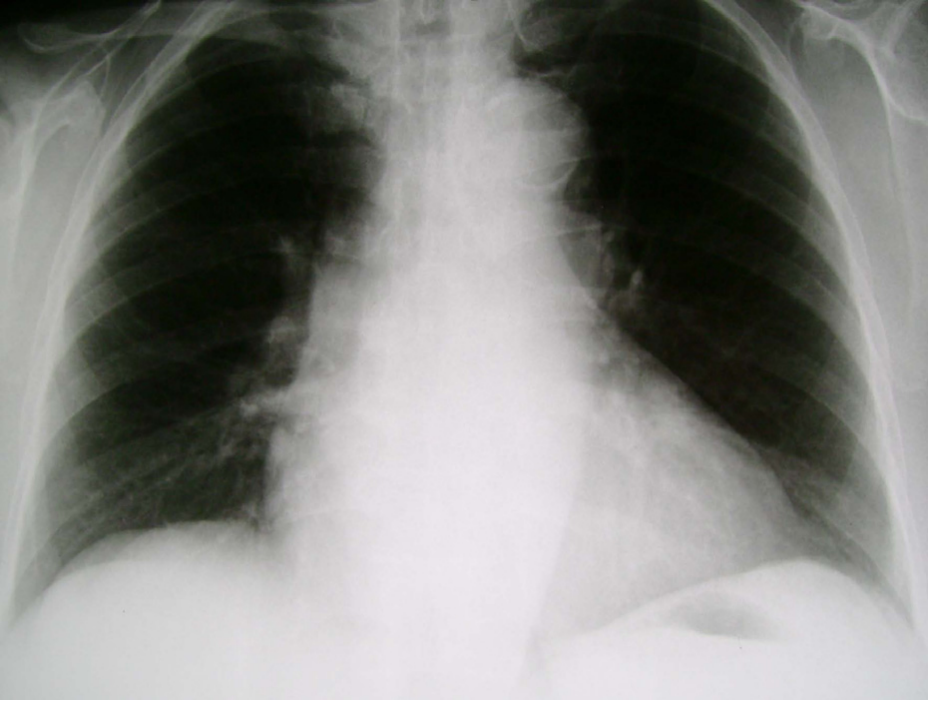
Upright posteroanterior chest radiograph just before the patient's discharge. No subdiaphragmatic free air is noted bilaterally.
